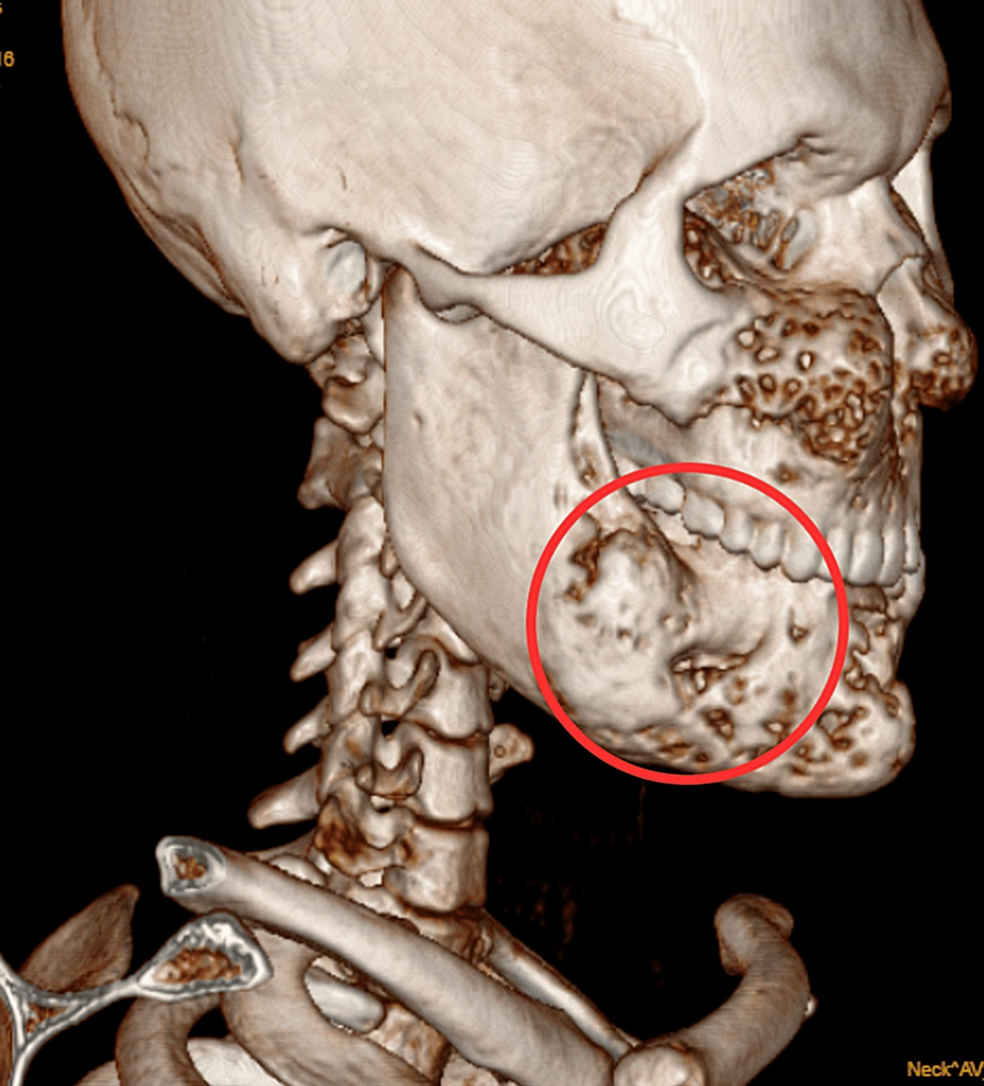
Patients computed tomography (CT) scan Red circle denotes the expansion of the bony calvarium of mandibular body.
